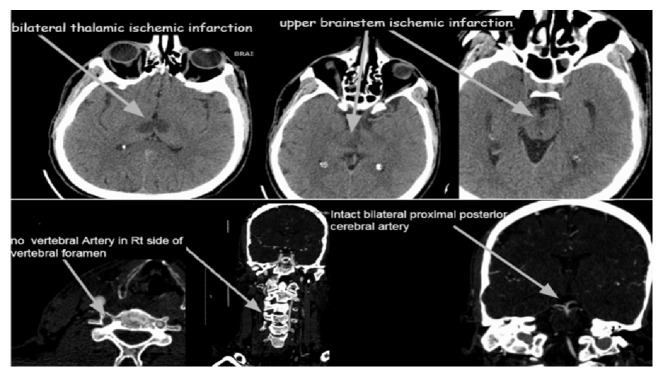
Brain and cervical CT Angiography (3rd day Post Op) revealed bilateral thalamic with upper brainstem ischemic infarction, with total occlusion of right vertebral artery from its origin and patent bilateral carotid artery with a normal flow.
